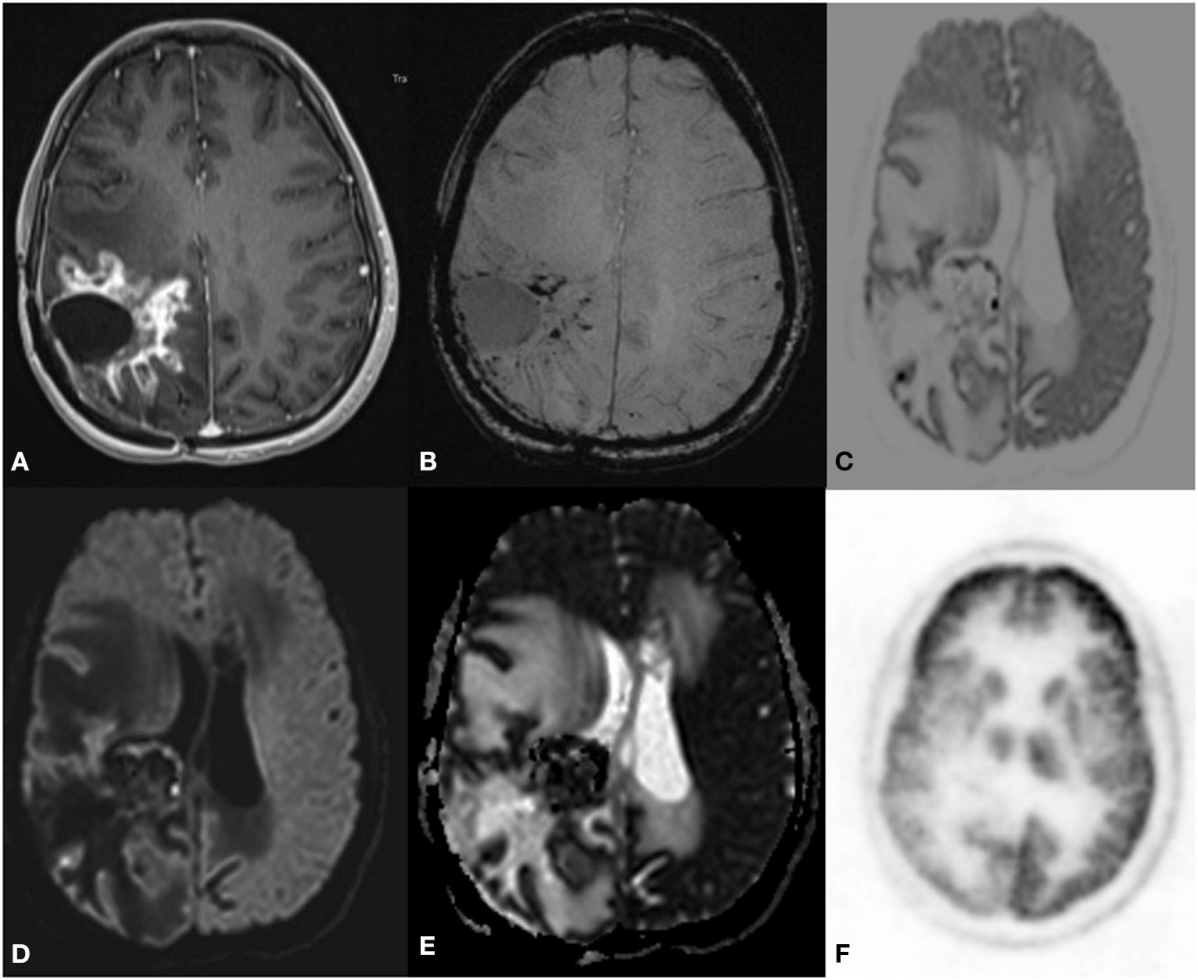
A 34 year old female postoperative case of anaplastic clear cell Ependymoma, 7 year follow up MRI F-18 choline PET axial images SWI (B) postcontrast T1 (A) Inverted DWI (C) DWI b2000 (D) ADC (E) NAC PET (F) showed a Swiss cheese and nodular enhancement lesion in the right parietal region. The lesion shows DWI restriction with hypermetabolism on PET. There is a match on the inverted DWI image and the NAC PET image, which is suggestive of recurrence.
